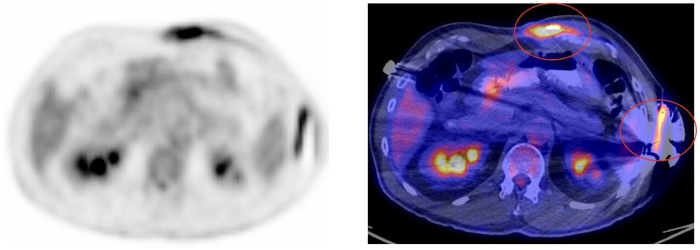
Attenuation-corrected fluorodeoxyglucose–positron emission tomography/computed tomography image showing focal radiotracer uptake localized to the extravascular implantable cardioverter-defibrillator generator and the distal portion of the lead (*red circles*).
